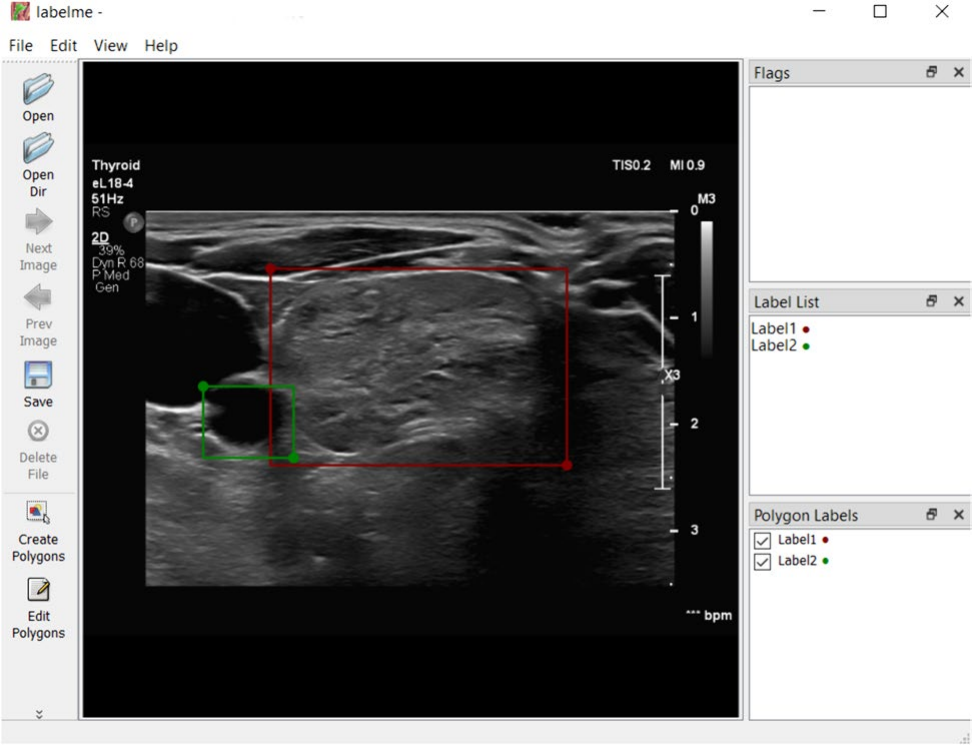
Bounding box generation by the “Labelme” software—two-point coordinates selected on an ultrasound frame (the green and red dot) are used to generate 2D rectangular boxes. The boxes’ center coordinates and dimensions in two axes are saved to be used as the “region of interest” or as labels for object detection tasks
